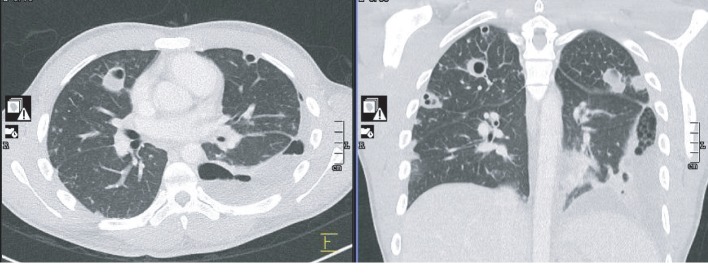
Repeat chest computed tomography (CT) on hospital day twelve (12) showing more pronounced cavitary lesions and moderate loculated left lower lobe pleural effusion.
